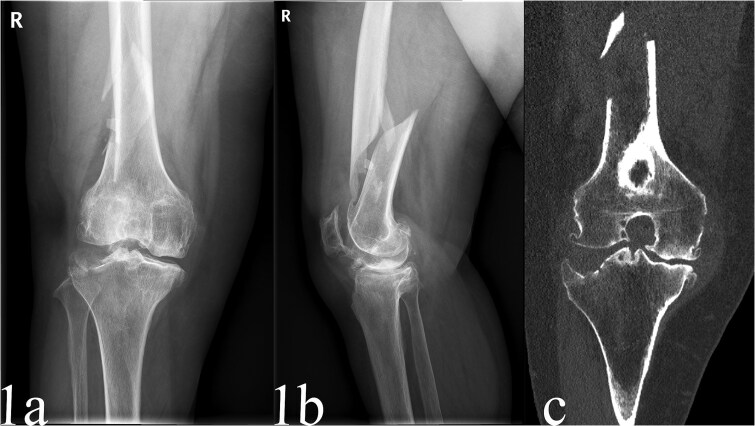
Preoperative anteroposterior radiographs of the case, sustained a displaced spiral fracture of the distal femur (AO/OTA type A1.2) and knee OA (Kellgren-Lawrence grade IV) (a and b). CT scans knee OA (Kellgren-Lawrence grade IV) (c).
